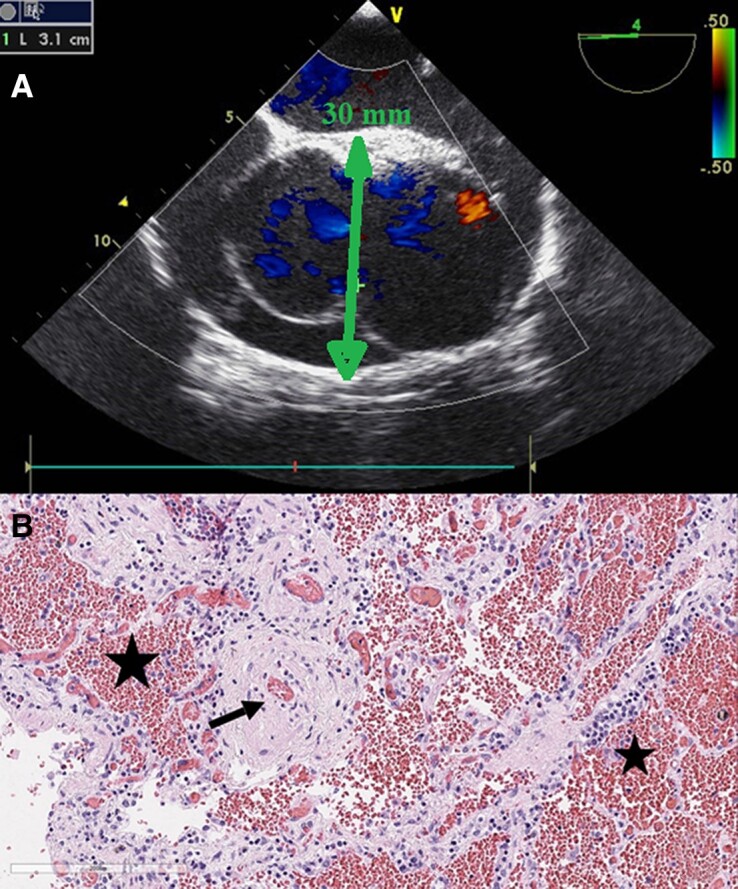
Patient 1. (*A*) Transoesophageal echocardiography at 29 5/7 weeks: ‘Supravalvular location of aortopulmonary junction up to 30 mm with balanced blood flow (truncus arteriosus type)’. (*B*) Concentric narrowing of the small-calibre pulmonary artery lumen due to wall fibrosis (arrow), intraalveolar haemorrhage as a demonstration of disseminated intravascular coagulation syndrome (asterisks), ×200 increase.
